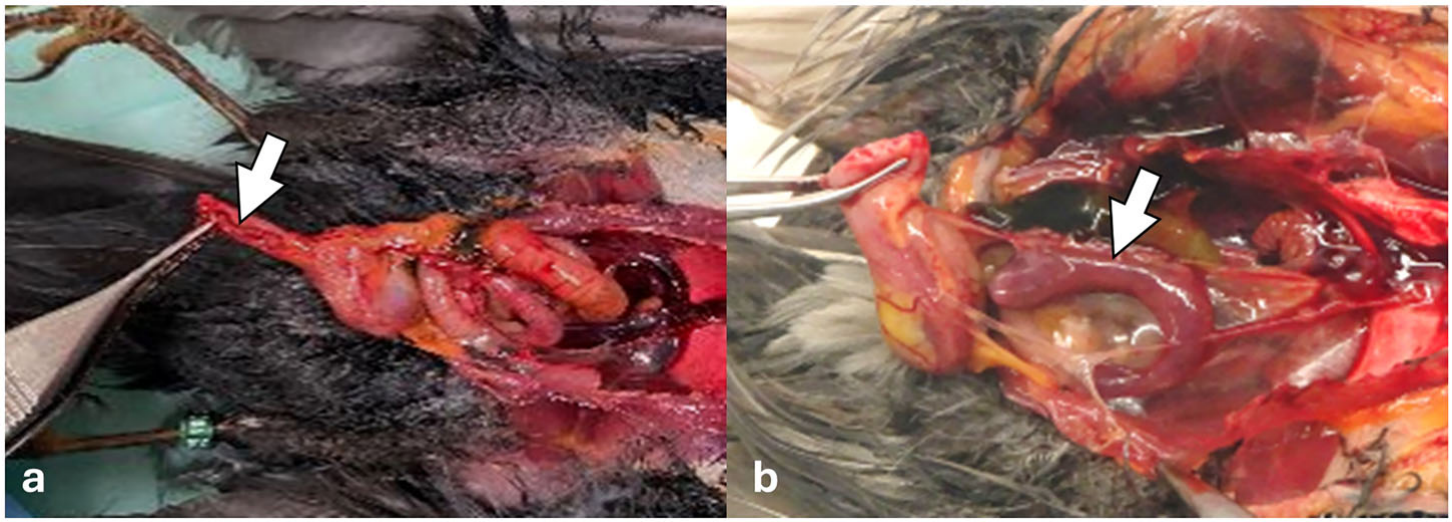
USUV-associated macroscopical lesions in representative control and infected blackbirds. Comparison of a a normal-sized spleen (arrowhead) in a control blackbird compared to b an enlarged spleen (arrowhead) in an infected blackbird.
